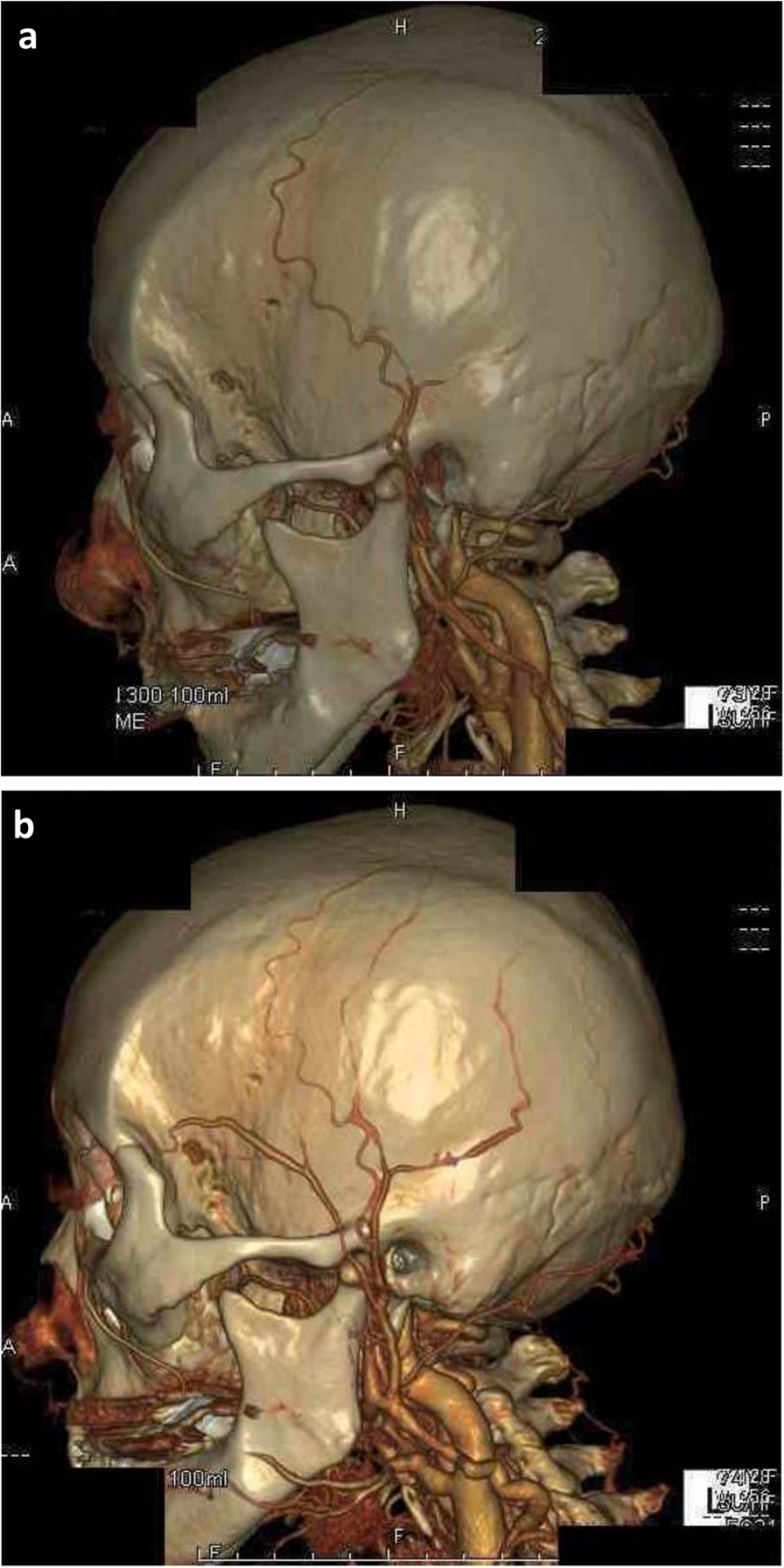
a Three-dimensional computed tomography angiography image of Case 2 before immunosuppressive therapy. b Three-dimensional computed tomography angiography image of Case 2 after therapy. Image (a) shows stoppage of the temporal artery, whereas (b) shows opening of the temporal artery
